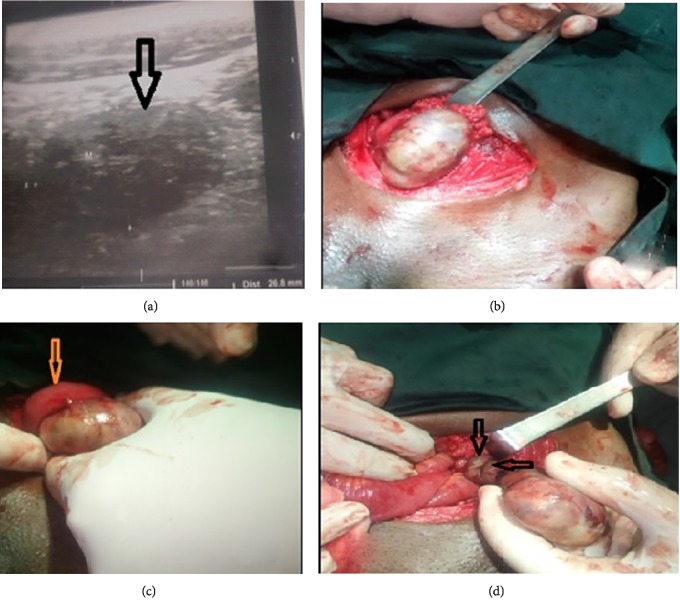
(a) Ultrasound (left lateral vesical mass); (b) mass of externalised testicular appearance; (c) intestinal loop adherent to the mass; (d) pedicle twisting.
